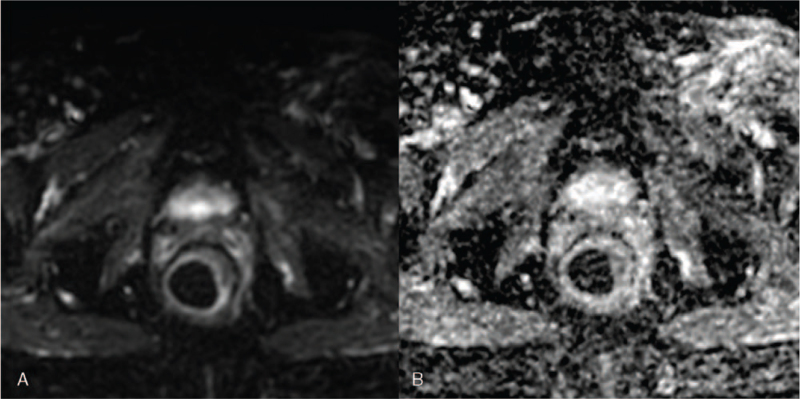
Diffusion-weighted magnetic resonance images performed 45 days after treatment. b = 1000 s/mm^2^ (A) and apparent diffusion coefficient map (B). Complete response: no evidence of suspected regions of water diffusion restriction.
